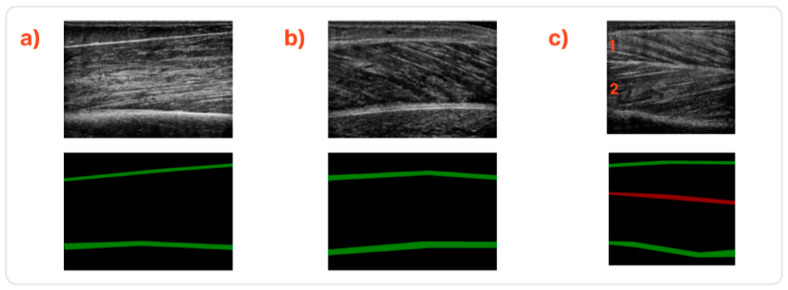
Sample ultrasound images along with their annotations. (a) Sample from the BB muscle, (b) from the GCM muscle, and (c) the TA muscle. The TA muscle comprises two compartments divided by the central fascia in the middle. These are depicted as 1 and 2.
